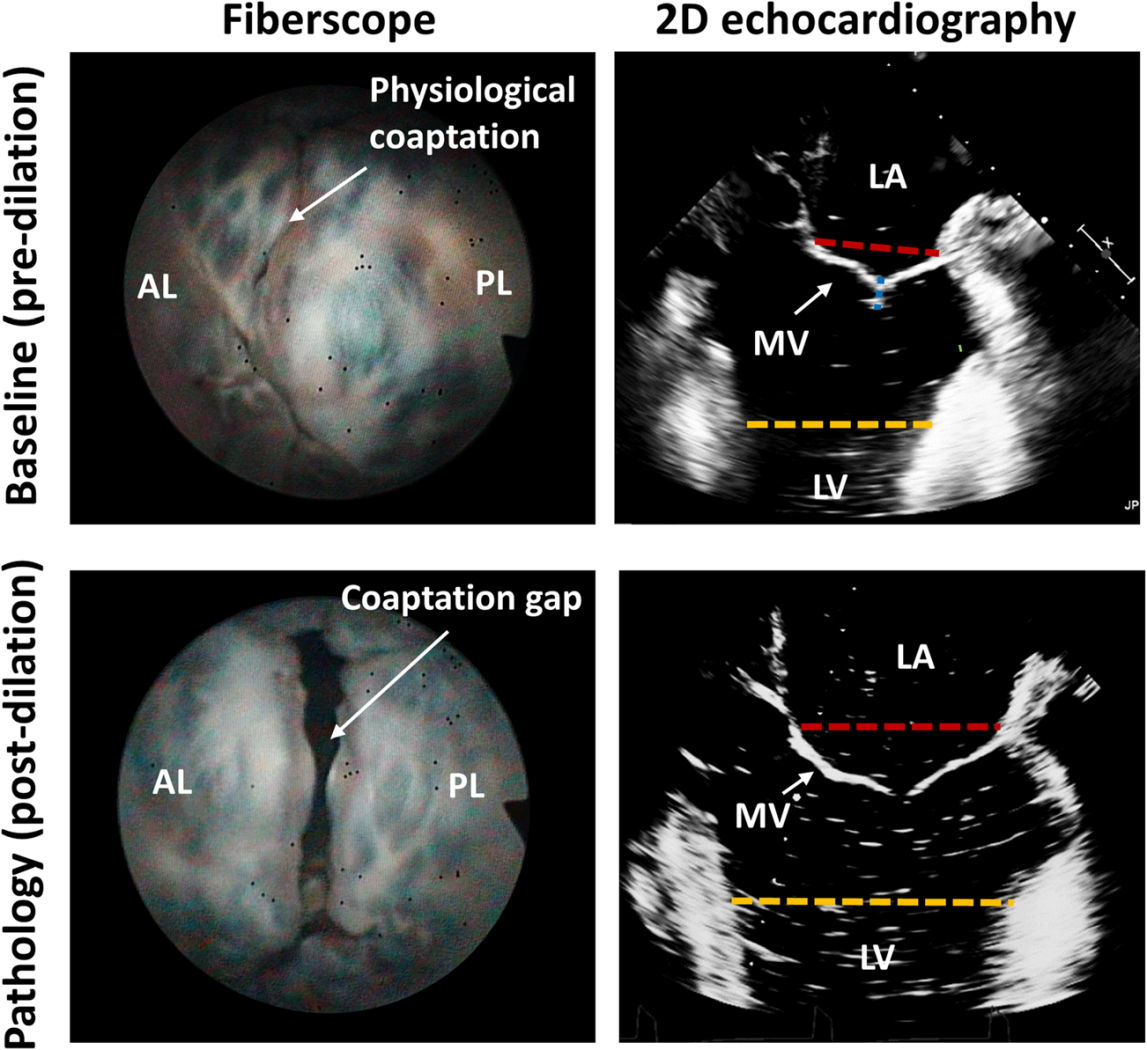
Dilation protocol. Mitral valve views from fiberscope (atrial view, left) and 2D echocardiography (TEE mid esophageal long axis like view, right) before (upper row) and after (lower row) application of the dilation protocol. Red dash line, antero-posterior distance; blue dashed line, coaptation length; orange dashed line, left ventricular diameter. AL = anterior leaflet, PL = posterior leaflet, MV = mitral valve, LA = left atrium, LV = left ventricle
